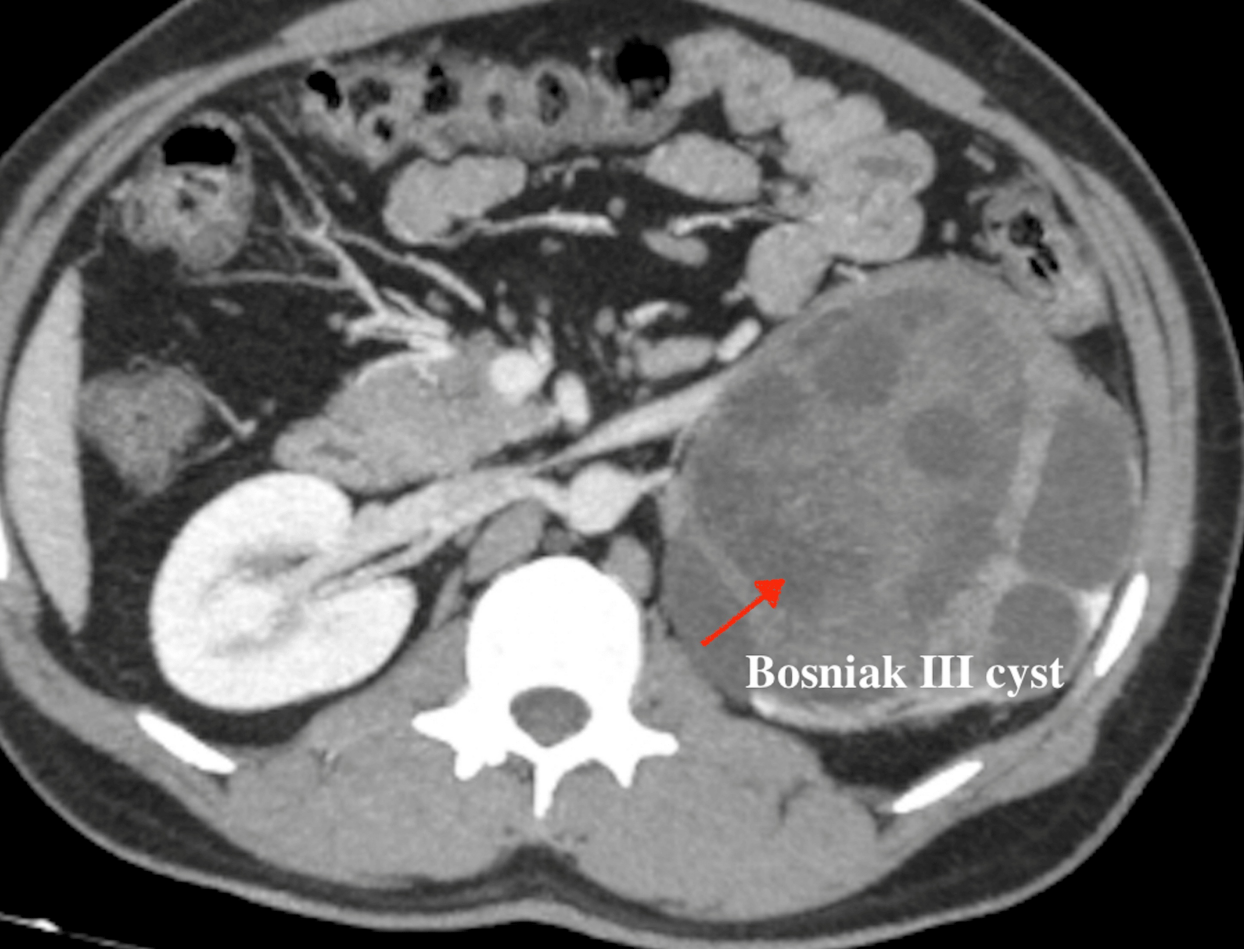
Bosniak type 3 cyst in the left kidney on the computed tomography scan.
